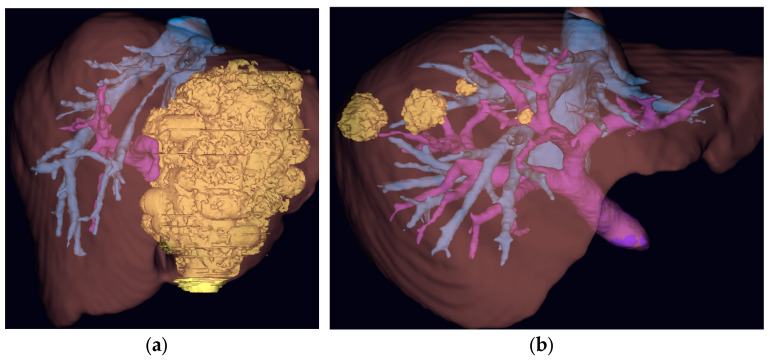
Images from HoloCare© Studio for liver surgery planning, 3D reconstructions of CRLMs using portal venous phase CT images (blue: hepatic veins; purple: portal veins; yellow: CRLM). (a) Single large CRLM threatening FLR; (b) multiple CRLMs in close proximity to hepatic and portal veins.
